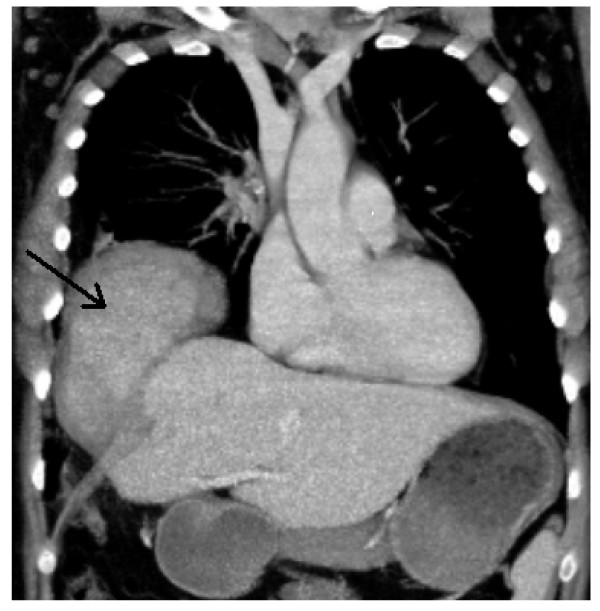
Computed tomography of the thorax and abdomen. At the sagittal section, a black arrow indicates the right lobe of the liver in the thorax cavity.
